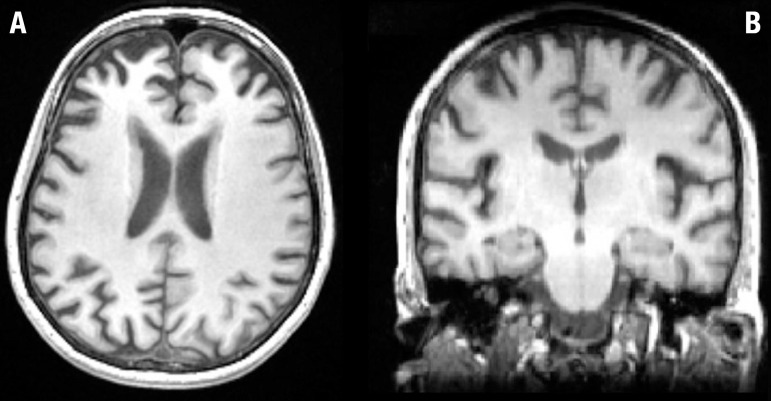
Brain MRI from patient TA, 48 year-old woman. [A] Axial slice: mild
